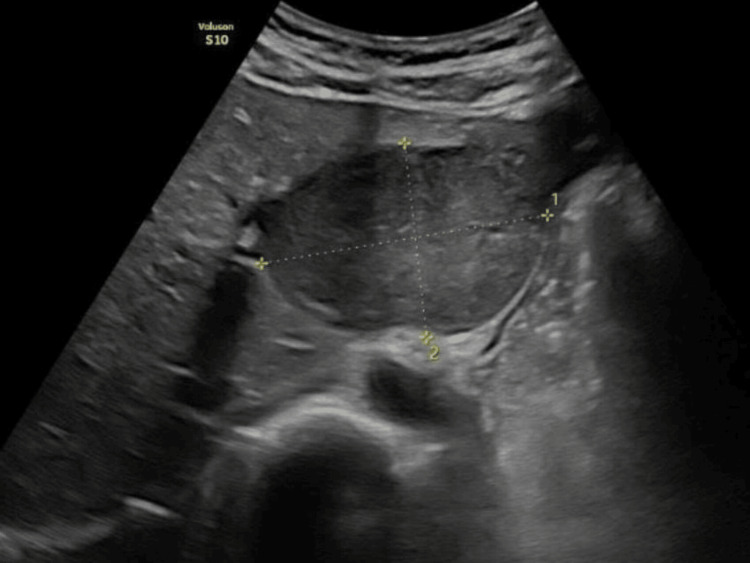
Abdominal ultrasonography demonstrating a relatively homogeneous hypoechoic mass in the left hepatic lobe, with a well-defined capsule and clear demarcation from the surrounding liver parenchyma.
